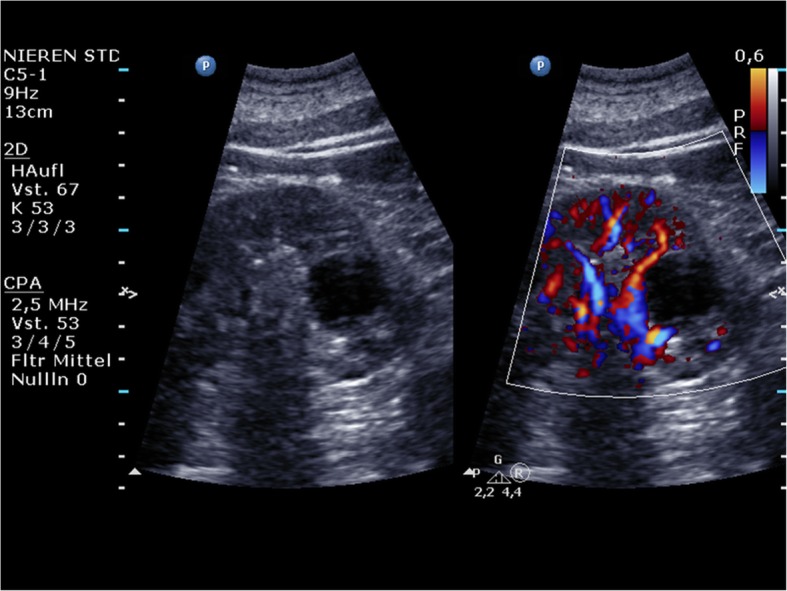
Philips Healthcare CX50: A renal cyst (left) that shows thin walls without any septa, calcifications, solid components, or contrast enhancement. There is no vascularisation of the cyst visible in colour Doppler mode (right)
